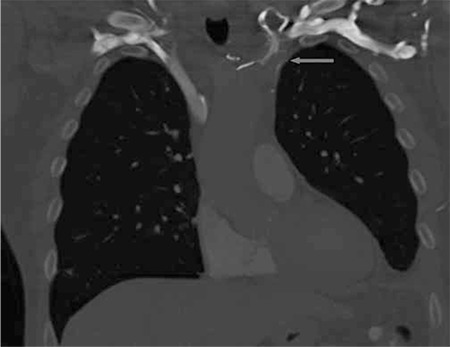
A coronal image reconstruction of a diagnostic CT scan with intravenous iodine contrast, made by the PET/CT. There is a contrast defect (gray arrow) in the proximal left innominate (brachiocepahlic) vein and formation of collateral flow, chages attributable to a thrombus. The latter was consequently confirmed by sonography.
